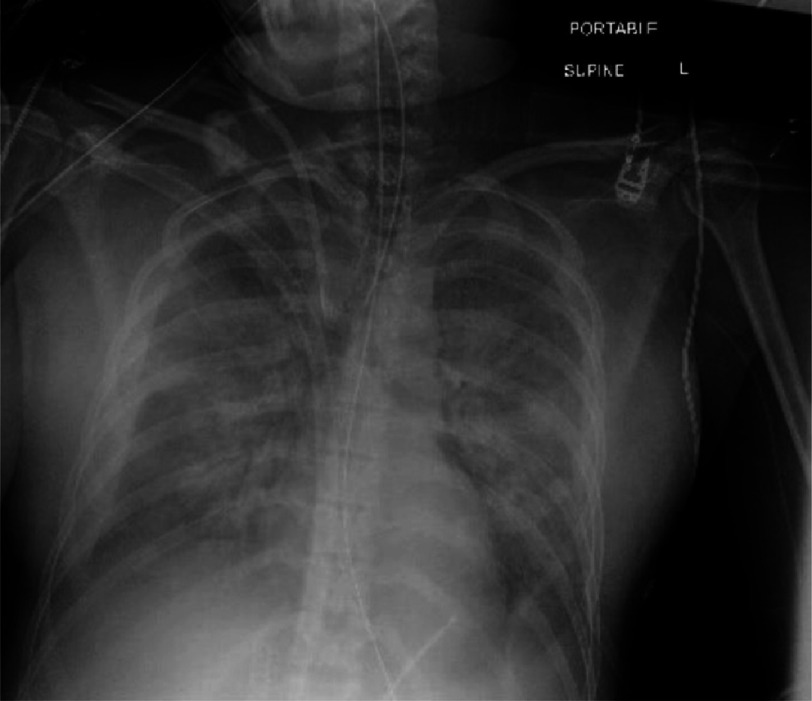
Chest X-ray showing signs of pulmonary edema.
